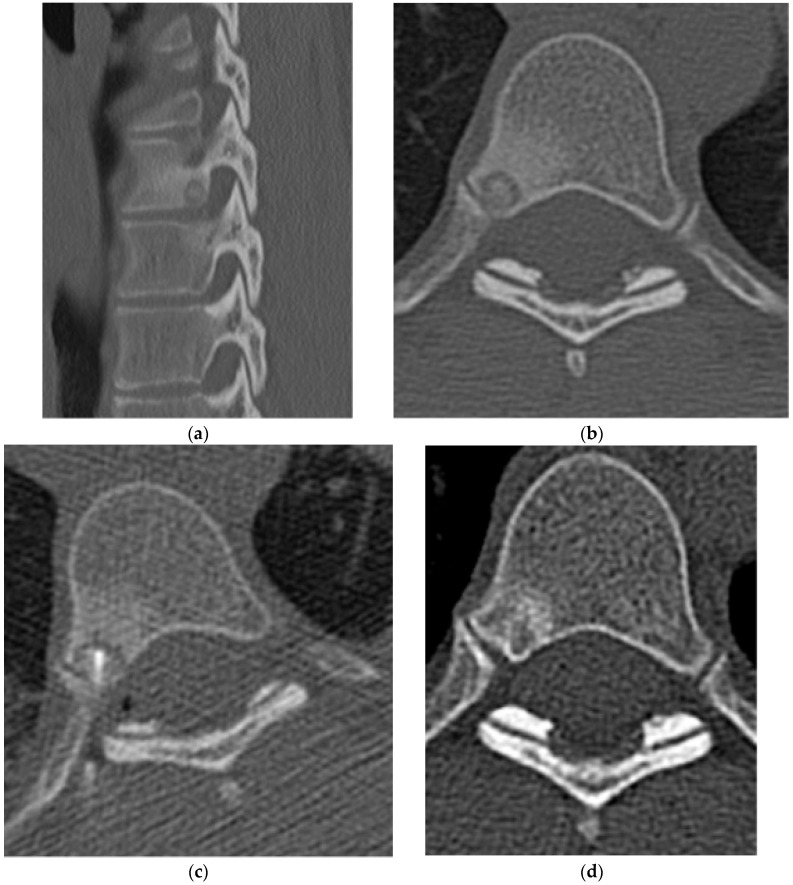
Osteoid osteoma. (a) Axial and (b) sagittal CT bone window demonstrate an osteoid osteoma in the right posterior aspect of a thoracic vertebral body with a central sclerotic nidus, surrounding lucency, and prominent surrounding edema. (c) Axial CT bone window image demonstrates a radiofrequency ablation probe in the nidus. (d) Follow-up images show resolution of nidus after radiofrequency ablation treatment.
